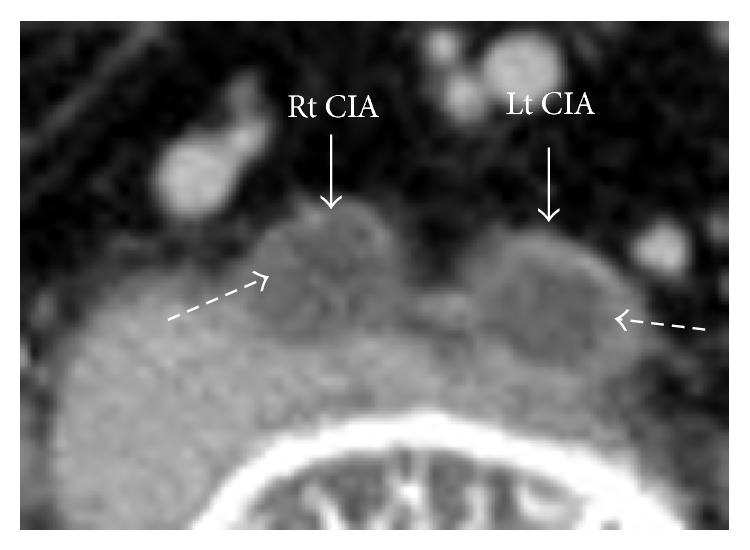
CT showed that the bilateral common iliac arteries were occluded with thrombus. The high density area was observed in low density area (dotted arrows).
